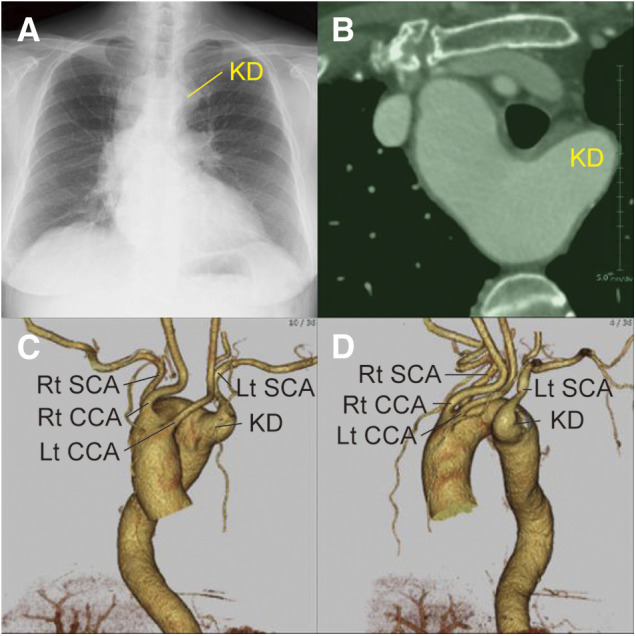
Fig. 1 Preoperative imaging studies. (A) Chest X-ray. (B–D) CT images. CT: computed tomography; KD: Kommerell diverticulum; Lt CCA: left common carotid artery; Lt SCA: left subclavian artery; Rt CCA: right common carotid artery; Rt SCA: right subclavian artery
